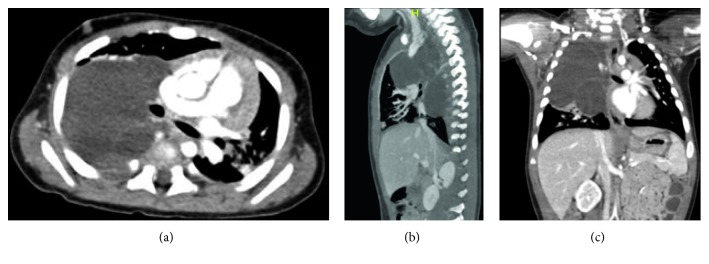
Contrast enhanced computed tomography (CT): a large multicystic lesion in the right hemithorax with mediastinal shifting. The bronchus and azygous are not affected.
